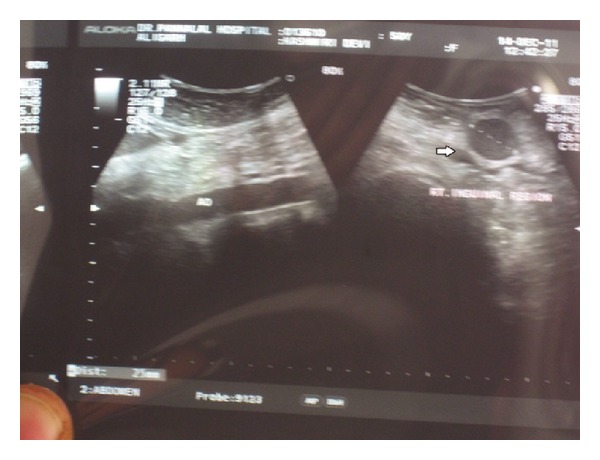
Ultrasound of enlarged right inguinal lymph node, 2.5 × 2 cm.
